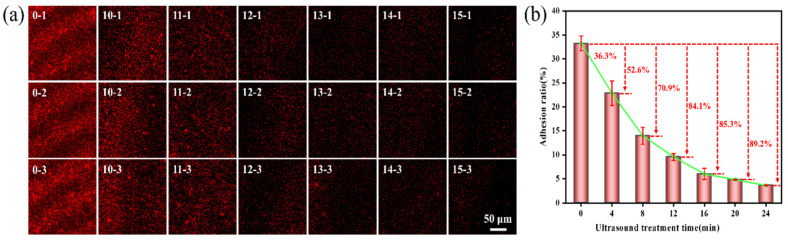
Effect of ultrasonic time on adhesion behavior of *Pseudoalteromonas*. (a) CLSM images of *Pseudoalteromonas* under different ultrasonic treatment times: 0–1~0–3 (0 min); 10–1~10–3 (4 min); 11–1~11–3 (8 min); 12–1~12–3 (12 min); 13–1~13–3 (16 min); 14–1~14–3 (20 min); 15–1~15–3 (24 min). (b) Curve of adhesion rate of *Pseudoalteromonas* with ultrasonic treatment time.
